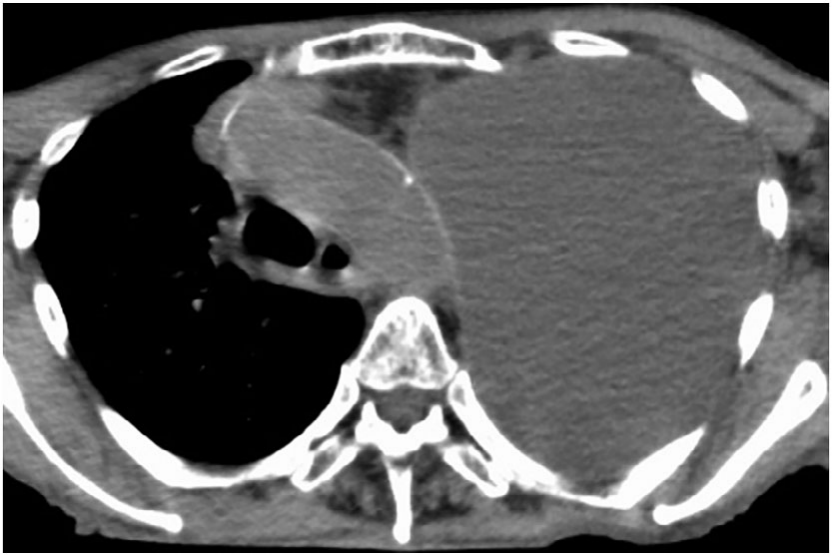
The computed tomography scan of the chest shows large left‐sided pleural effusions.
